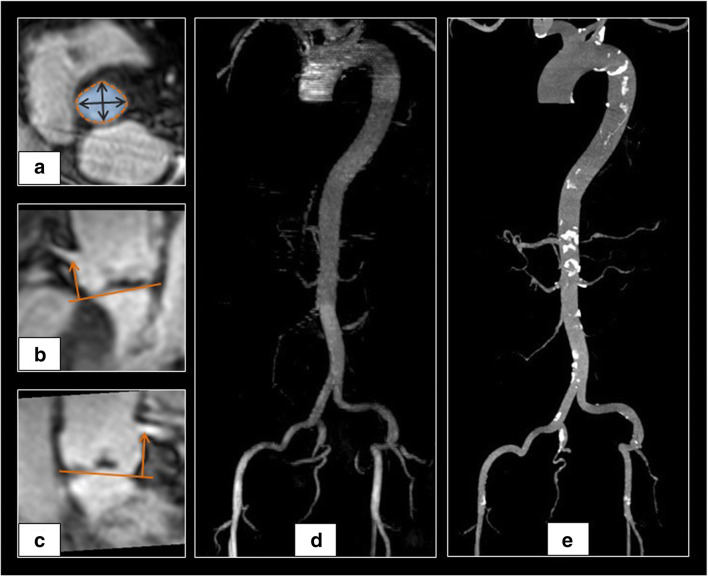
Imaging example of non-contrast 3D “whole heart” MRI for (a) aortic annulus measurements at the hinge point plane, the (b) right coronary artery, and the (c) left main artery ostial height measurement in the longitudinal axis at a right angle to the hinge point plane. Representative maximum intensity projection of aortoiliofemoral QISS-MRA (d) and CTA (e) datasets
